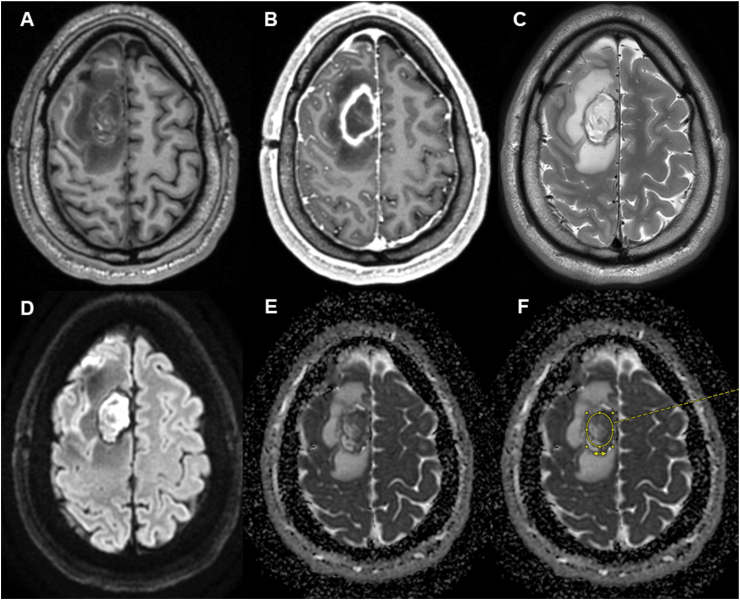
Representative magnetic resonance imaging (MRI) of a postoperative right frontal brain abscess extending into the subdural/intracerebral compartment following glioma resection. (A) Native T1-weighted image demonstrating an iso-/hypointense lesion within the postoperative resection cavity. (B) Contrast-enhanced T1-weighted image showing pronounced ring enhancement consistent with an encapsulated abscess. (C) T2-weighted image depicting a hyperintense cavity with surrounding edema. (D) Diffusion-weighted imaging (DWI) revealing marked diffusion restriction within the abscess cavity. (E) Corresponding apparent diffusion coefficient (ADC) map demonstrating low ADC values in the area of diffusion restriction. (F) ADC map with an exemplary elliptical region of interest (ROI) placed within the abscess cavity for quantitative ADC measurement. The ROI was positioned in regions of maximal signal intensity on the b1000 image and transferred to the co-registered ADC map, with anatomical verification using T2-weighted and DWI sequences, as described in the Methods section.
